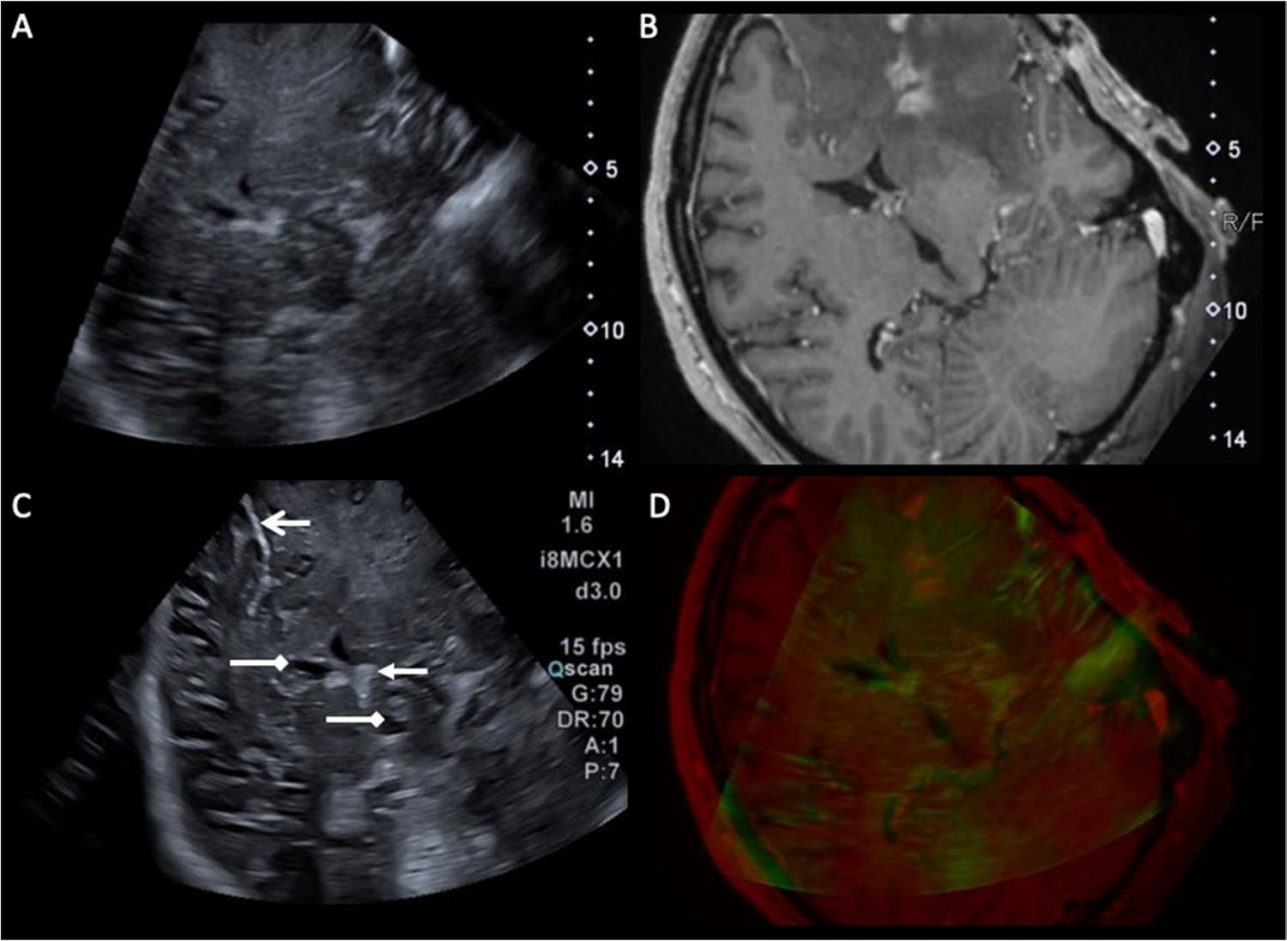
Example of navigated 3D ultrasound fused with MRI and useful anatomical landmarks. Navigated 3D ultrasound fused with MRI using rigid registration of anatomical features between a 3D ultrasound dataset (A) and the preoperative post contrast T1w navigation MRI (B). Live 2D ultrasound image (C) registered with the fused 3D US-MRI images (A,B) with the position of the probe tracked by an electromagnetic sensor. Overlay of the 2D ultrasound image on MRI showing quality of fusion (D), with the green mask representing hyperechoic structures on the US. Note the relatively poor sonographic definition of the tumour which is greatly augmented by fusion with the MRI. Useful anatomical landmarks for orientation labelled on (C): hyperechoic falx (open arrow head), lateral and 3^rd^ ventricles (top and bottom chevrons respectively) and choroid plexus in the lateral ventricle (closed arrow head)
